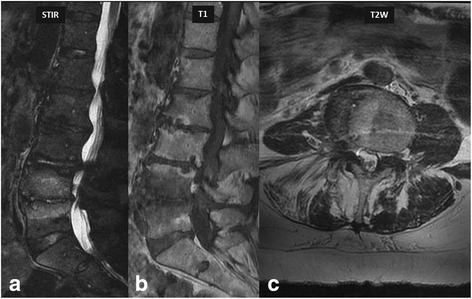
An example of the Modic changes related to degenerative disease in a 68-year-old farmer. Note the Type I Modic changes at L4–5 endplates showing hyperintense signal on STIR image (a) and hypointense signal on T1-weighted image (b). Also note the absence of any surrounding paravertebral edema or psoas involvement (c), differentiating these Modic changes from vertebral osteomyelitis
